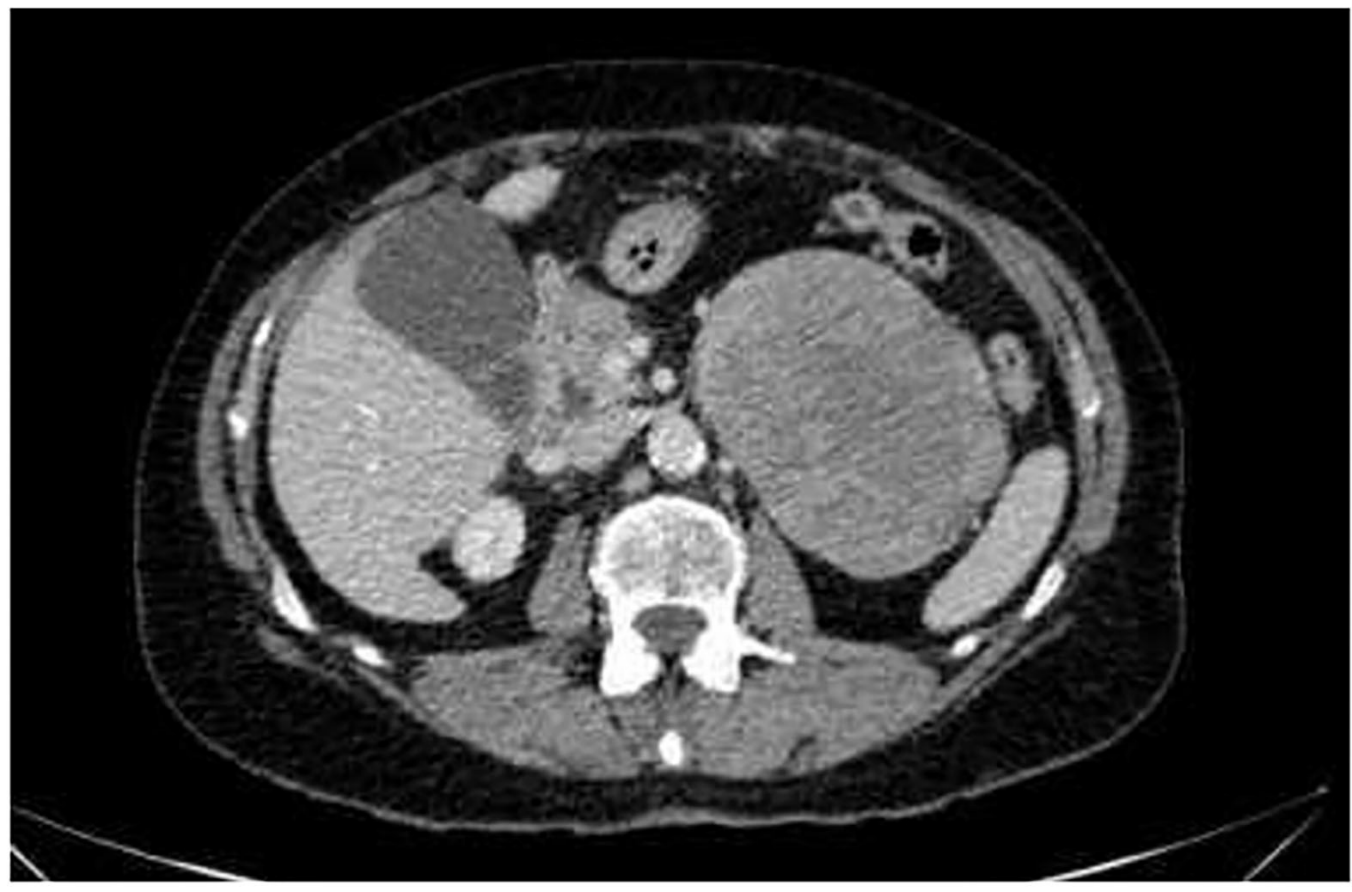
CT-scan of the abdomen. Axial CT scan of the abdomen reveals a large abdominal mass measuring 10 × 8 × 10 cm without lymphadenopathy.
